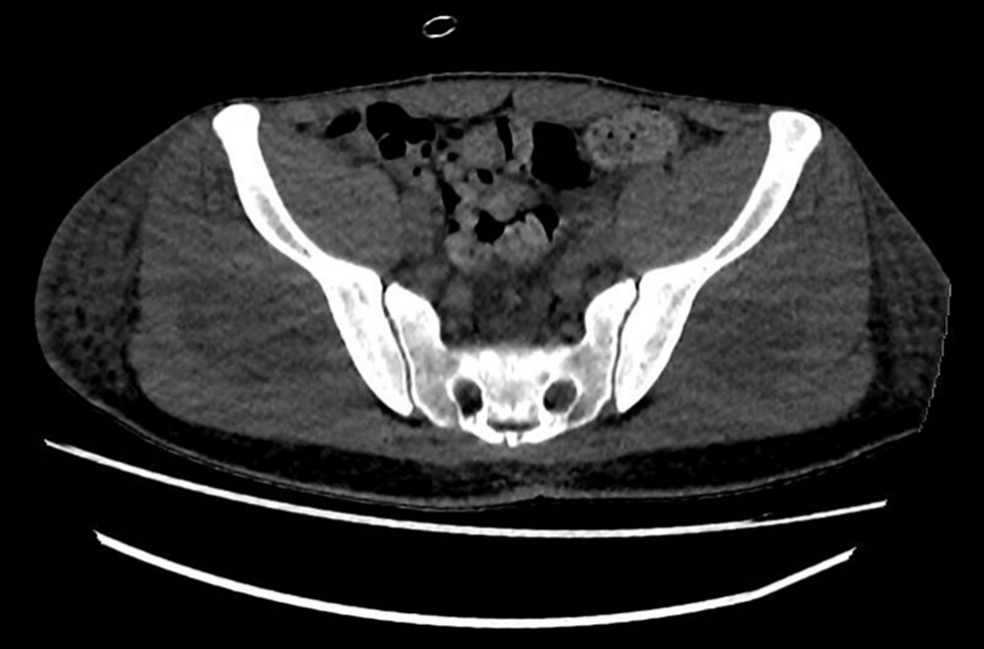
CT KUB showing swelling of the bilateral gluteus medius and minimus muscles with faint hypodense areas within, with overlying subcutaneous fat stranding and oedema CT KUB: computed tomography of the kidneys, ureters, and bladder.
